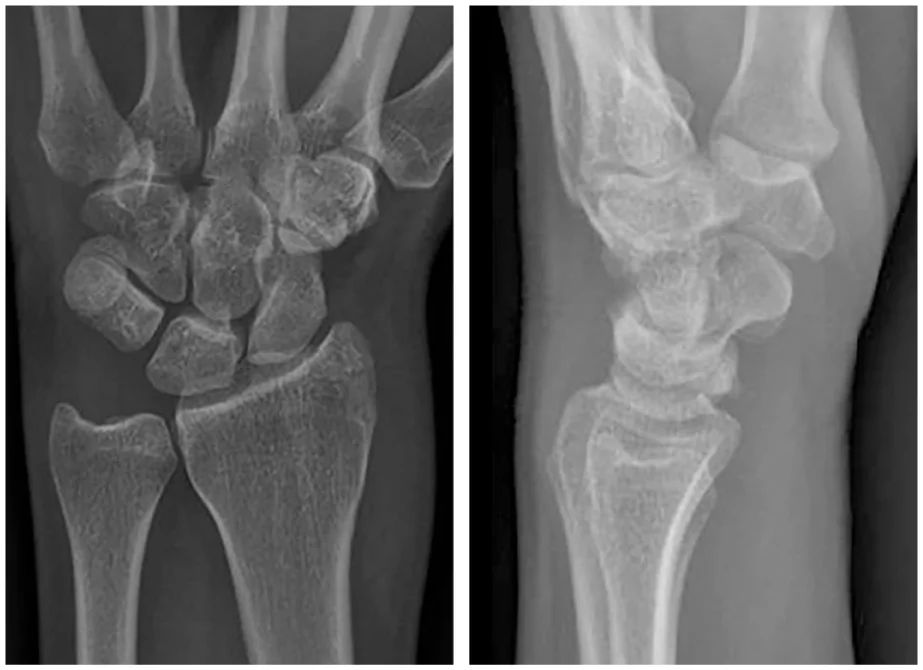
Initial posteroanterior and lateral radiographs of the left wrist. In retrospect, a small radial styloid/rim avulsion fragment is visible; radiocarpal incongruity is subtle on the lateral view.
